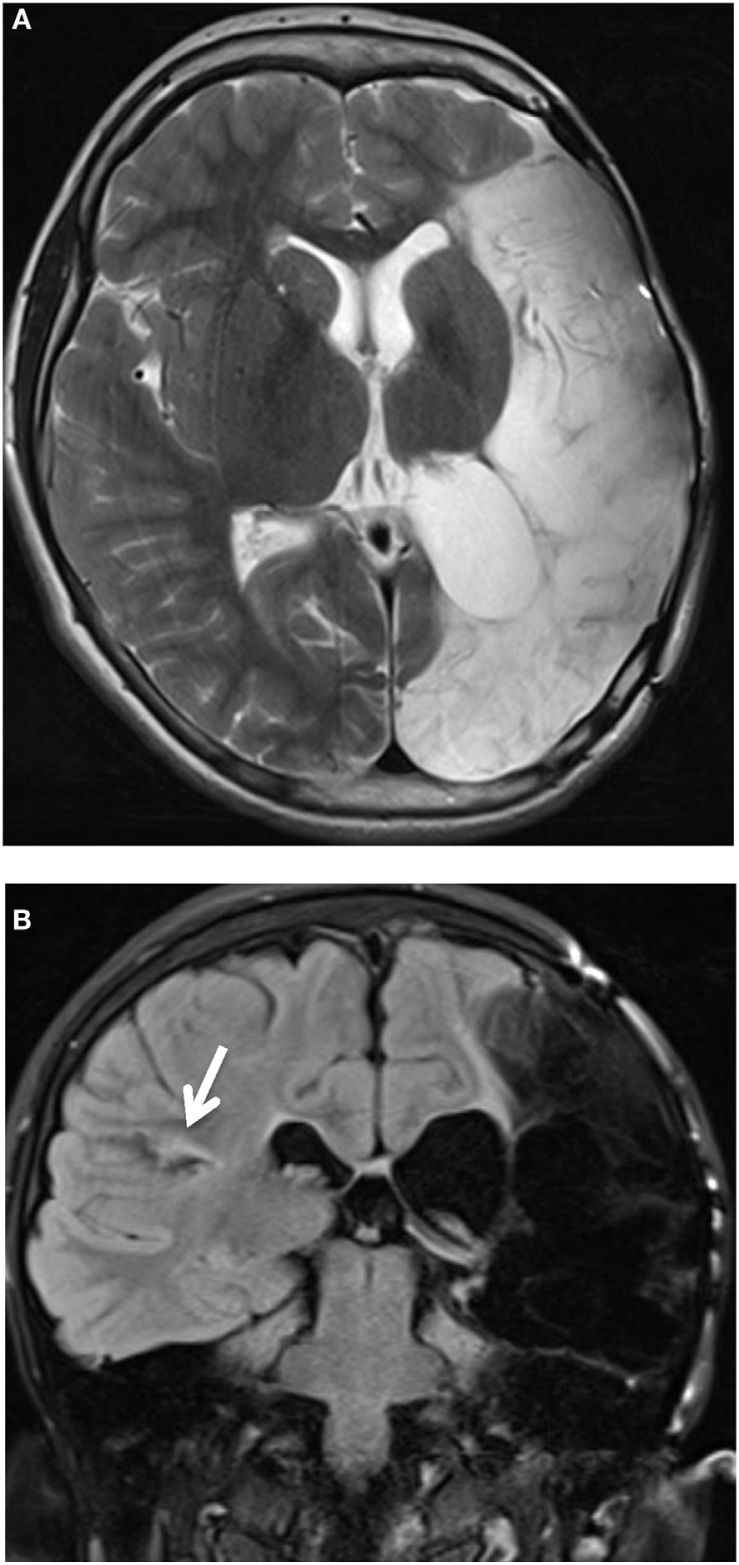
Axial T2-weighted image. (A) Presence of left craniectomy. Extensive mature injury mostly involving the left middle and posterior cerebral artery territories. Nearly all of the left temporal lobe was involved (not shown). (B) Coronal T2-weighted FLAIR image. Less extensive mature regions of cortical injury, best seen in the right posterior sylvian cortex (white arrow) and right parietal cortex. Symmetrical mature injury of the cerebellar hemispheres was also noted (not shown).
